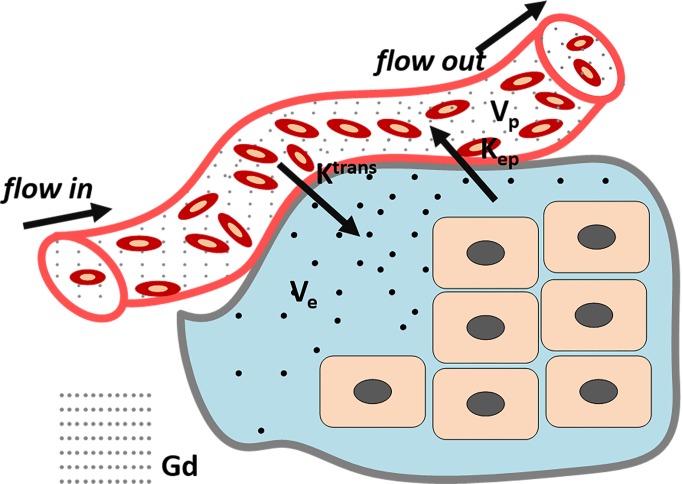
Tofts Model: classical DCE-MRI model (K^trans^: the transfer constant from vascular to extravascular extracellular space (EES); K_ep_: Rate constant between the EES and the blood plasma; V_e_: The fractional tissue EES (light green). V_p_: The fractional plasma volume (white)
